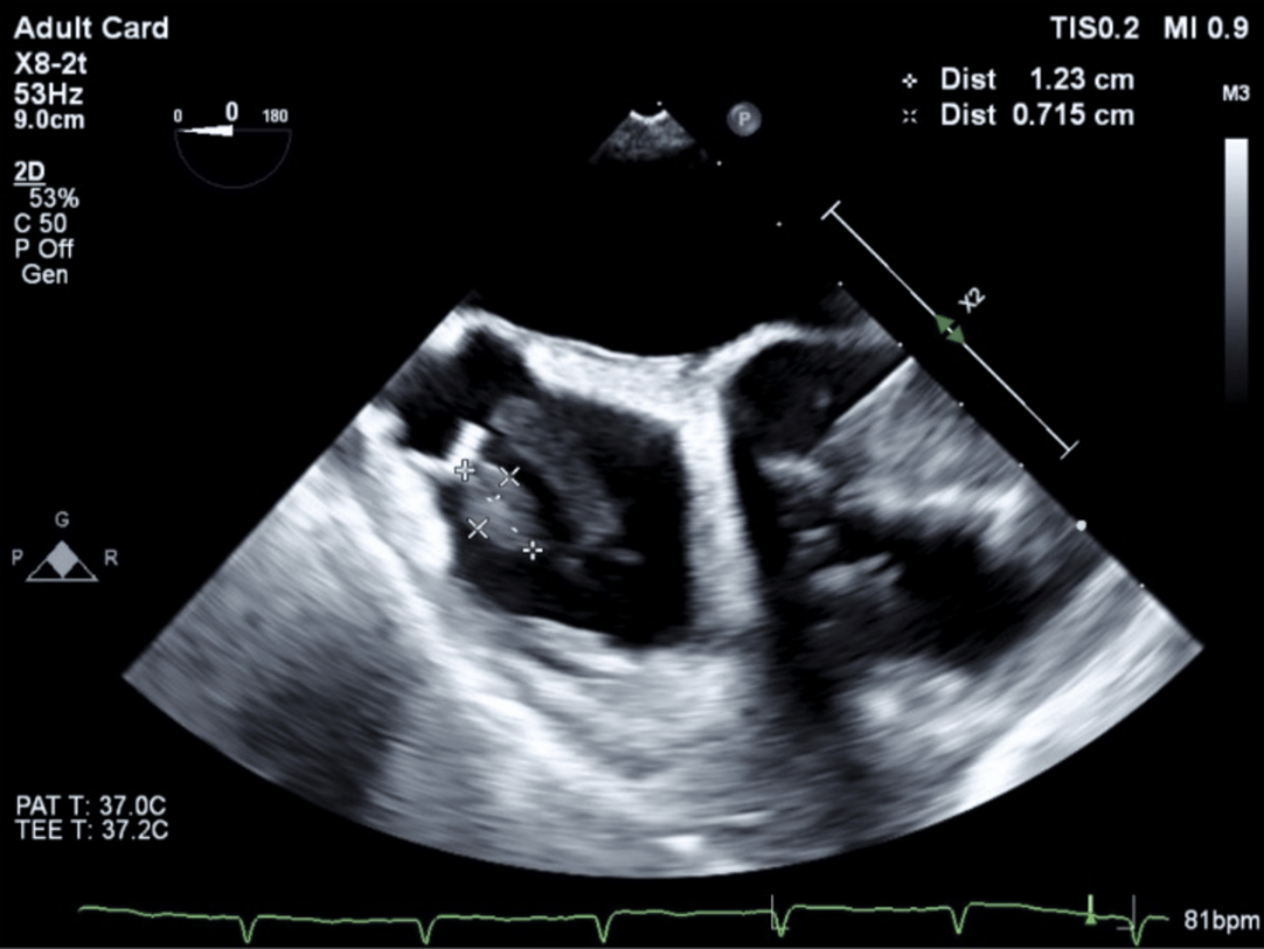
Transesophageal echocardiogram (mid-esophageal view) A large, mobile right atrial mass is seen measuring approximately 1.23 × 0.715 cm, consistent with vegetation attached to the catheter tip at the superior vena cava right atrial junction.
